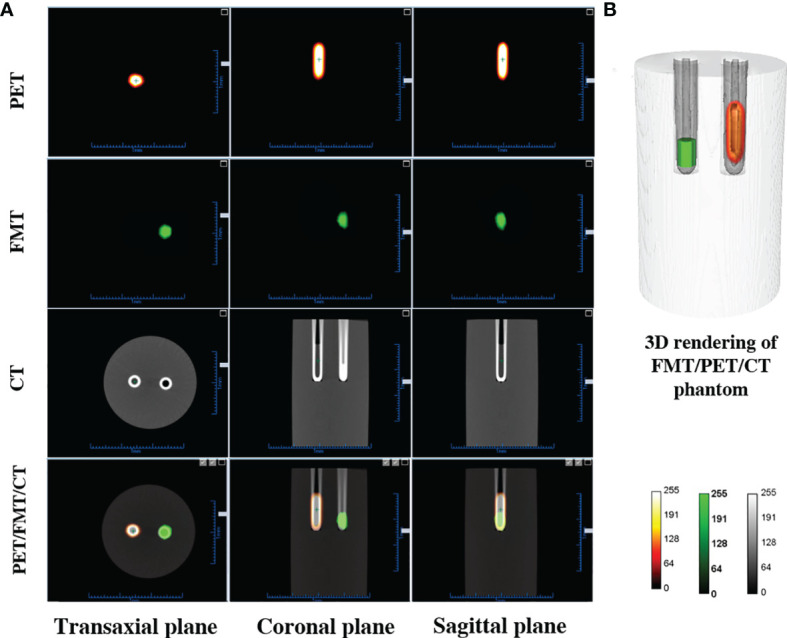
Results for the multi-modality imaging. (A) PET, FMT, CT, and tri-modality fusion images. (B) Three-dimensional rendering of fusion images demonstrating co-registration performance.
